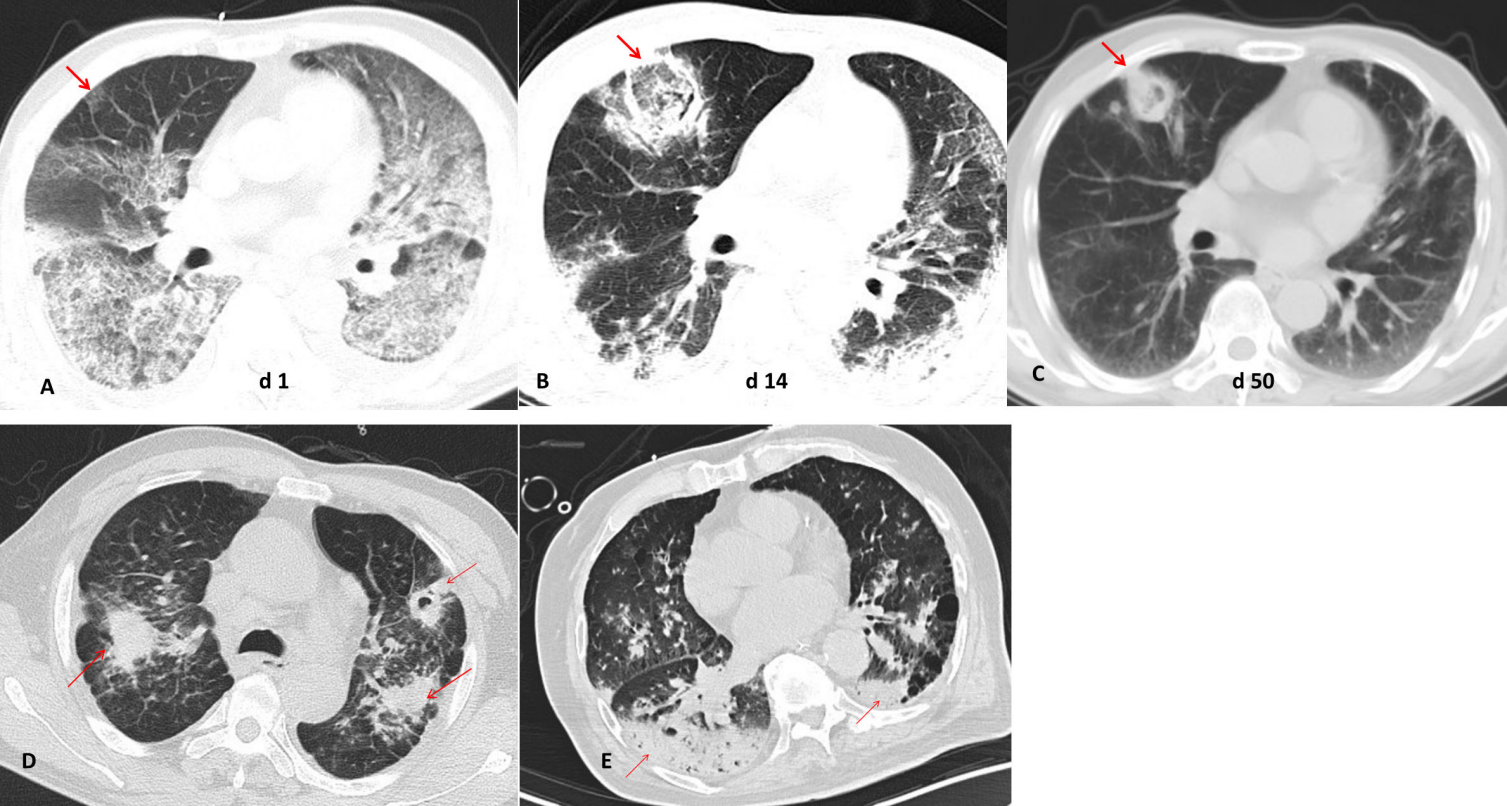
Different chest CT imagings in IAPA patients. (A) An IAPA patient’s right lung lesion initially presented as ground glass opacities, (B) then developed into subpleural wedge-shaped opacities, (C) and later became solid nodular opacities. (D) Multiple chest CT imaging forms such as cavities and consolidation shadows coexist in one IAPA patient. (E) Consolidation shadows with or without inflation sign in chest CT imaging of one patient. The red arrow indicates the lesion.
